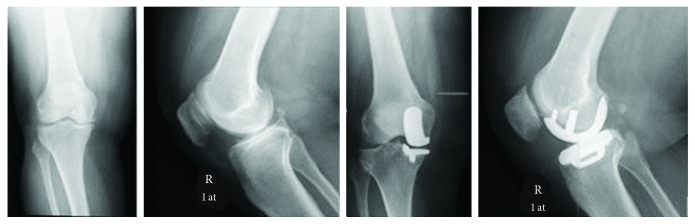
Preoperative and postoperative standing AP and lateral X-rays.
